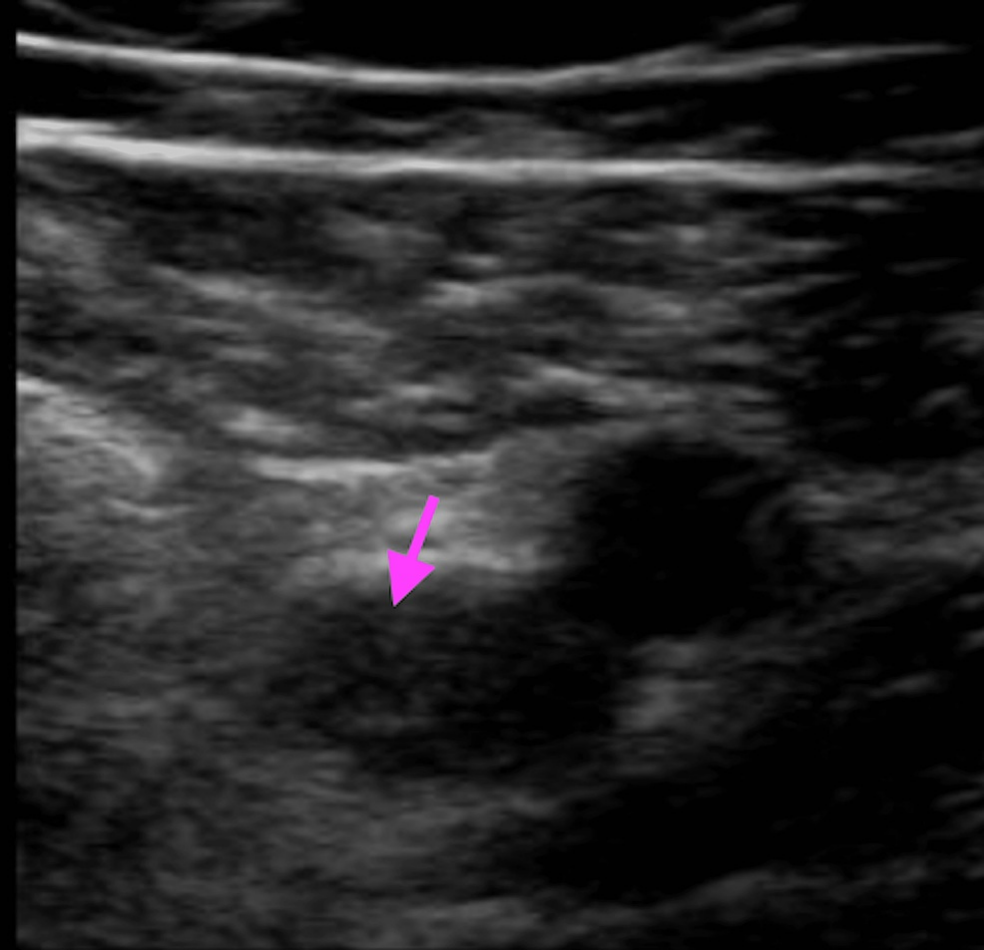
Occlusive deep vein thrombosis involving the right superficial femoral vein on ultrasound in Case 4 DVT: deep vein thrombosis
